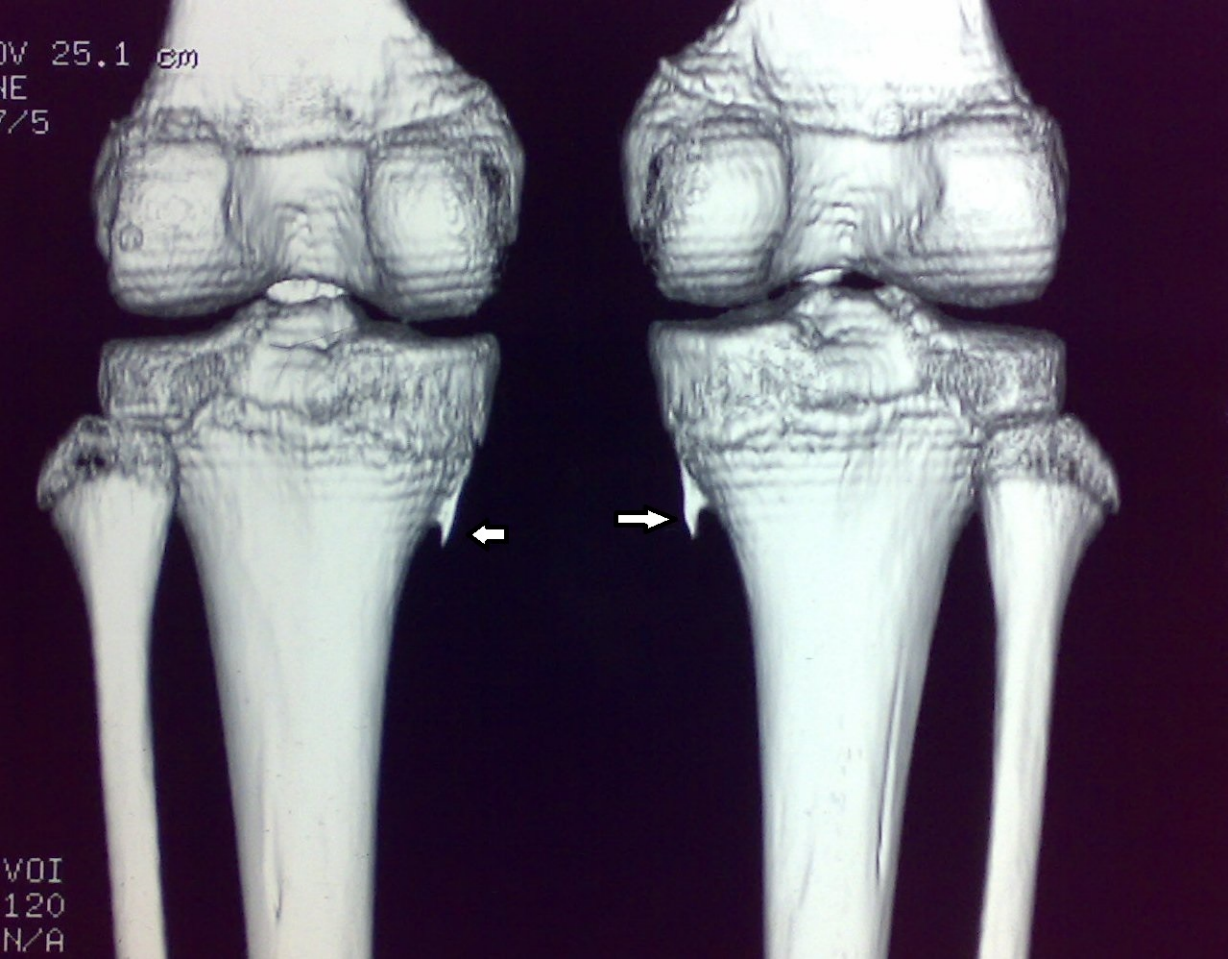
Case 1: Three-dimensional (3D) reconstruction CT scan of both knees Sharp “rose-thorn” like bony spurs (white arrows) arising from the medial proximal tibial metaphysis of both legs hanging down like an icicle.
